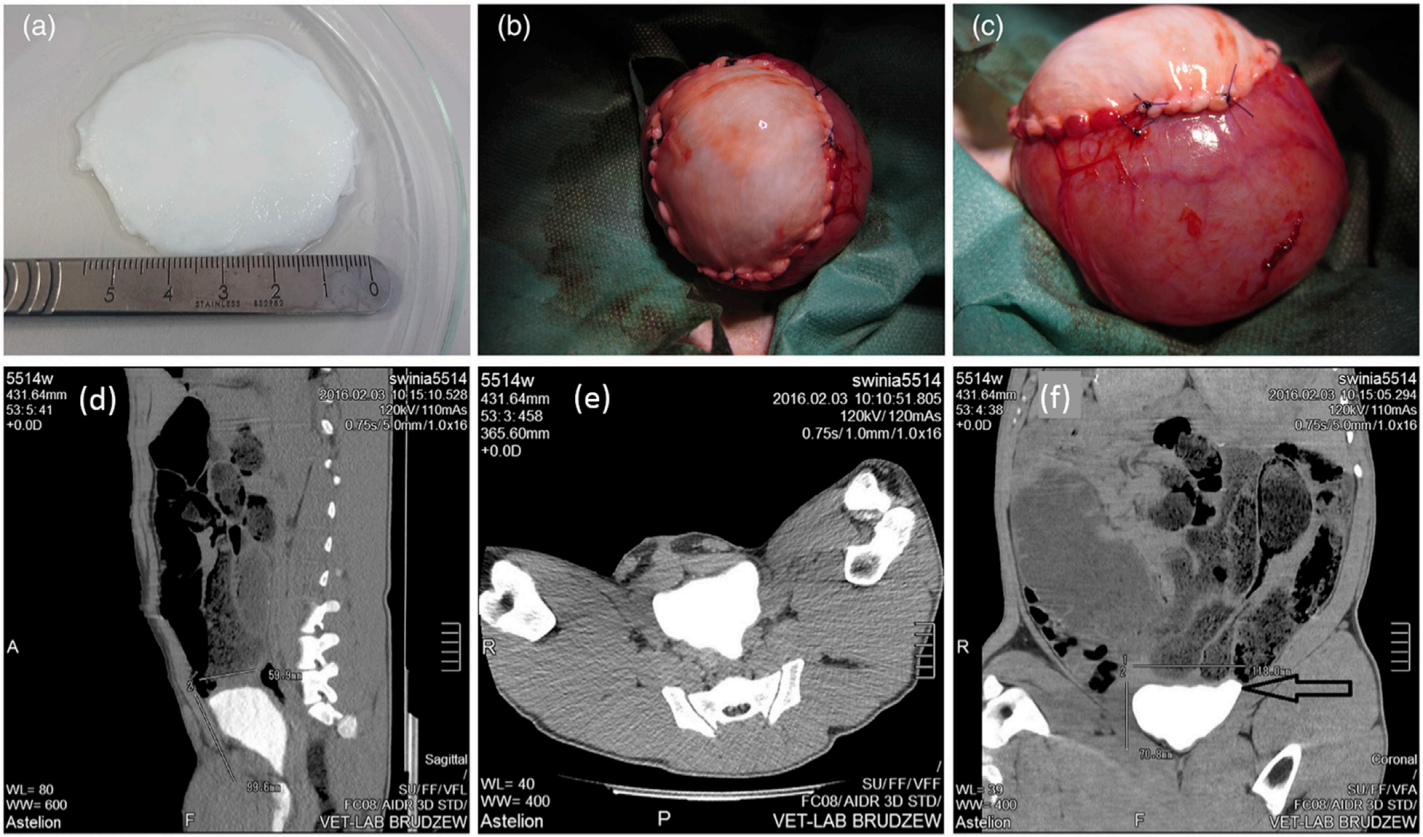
General observation of bladder acellular matrix (BAM) prepared for bladder reconstruction (A). Implantation of BAM into the site of bladder resection (B). The BAM graft was sutured with non absorbable sutures to the edge of the bladder resection (C). (D–F) Computed tomography images for 6-month follow-up after BAM implantation surgery. Adapted from (Pokrywczynska et al., 2022), Copyright 2021 John Wiley and Sons.
